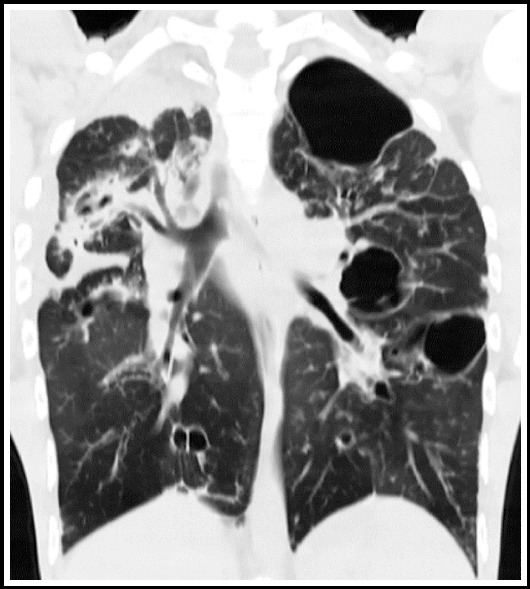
CT scan chest in lung window settings, shows traction bronchiectasis on the right along with patchy fibrosis. At least 3 lung cysts are seen on the left.
